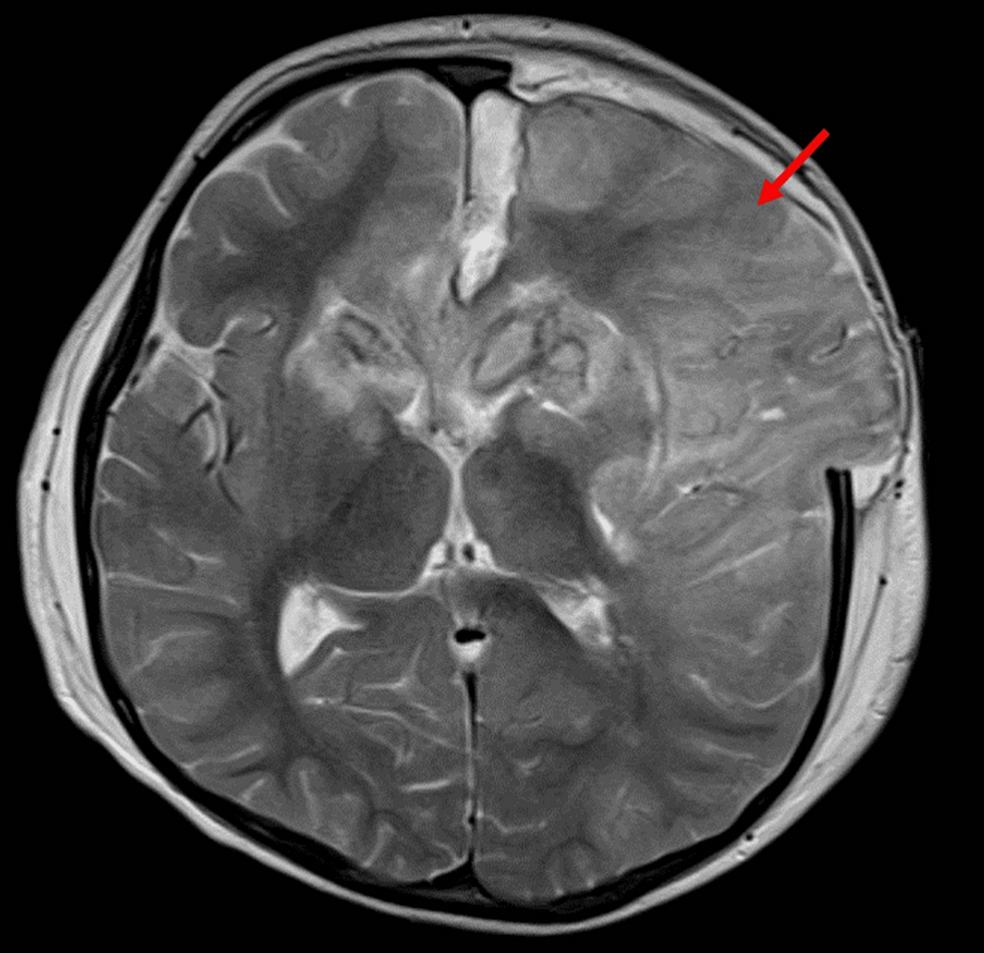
MRI of the brain Left hemisphere edematous changes with midline shift S/P left frontoparietal decompression craniectomy (red arrow). MRI: magnetic resonance imaging, S/P: status post
